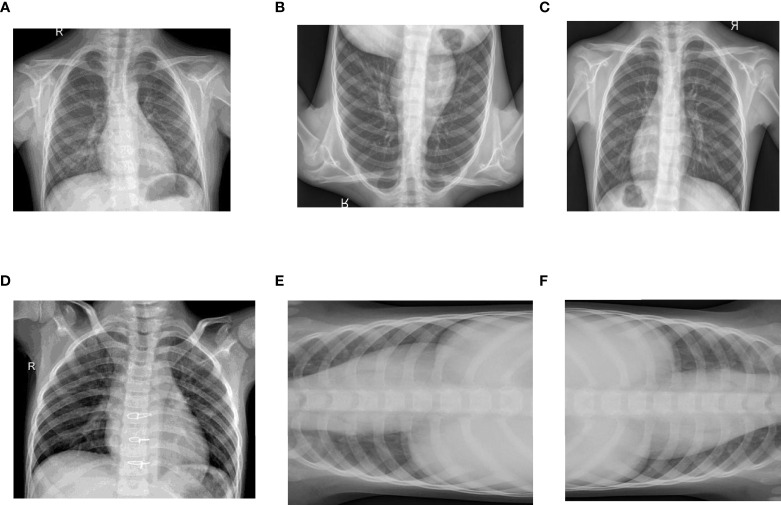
Data Augmentation of Images: (A) Original Healthy Chest X-ray, (B) Healthy Chest X-ray Flipped Vertically, (C) Healthy Chest X-ray Flipped Horizontally, (D) Original Pneumonic Chest X-ray, (E) Pneumonic Chest X-ray rotated 90 degrees left and (F) Pneumonic Chest X-ray rotated 90 degrees right.
